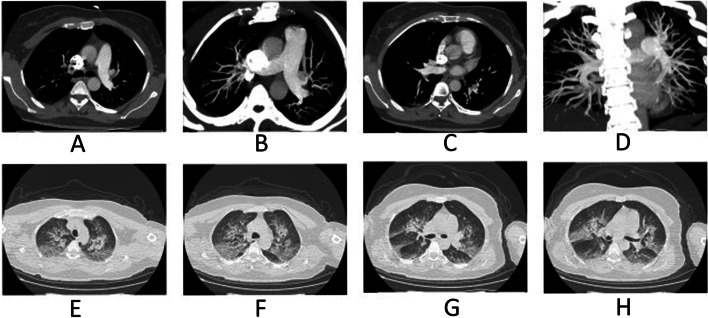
High altitude pulmonary edema (HAPE) in a 39-year-old boy who had dyspnea at rest and worsening cough for 3 days before admission. A-D Axial image of CT pulmonary angiogram showing thrombi as filling defects in right main pulmonary artery extending into its branch and in distal left pulmonary artery with extension into its superior branch. E-H Multiple lesions of ground glass opacity, patchy lesions and partial consolidation in the CT imaging were presented in the bilateral lung center along the bronchovascular bundle
